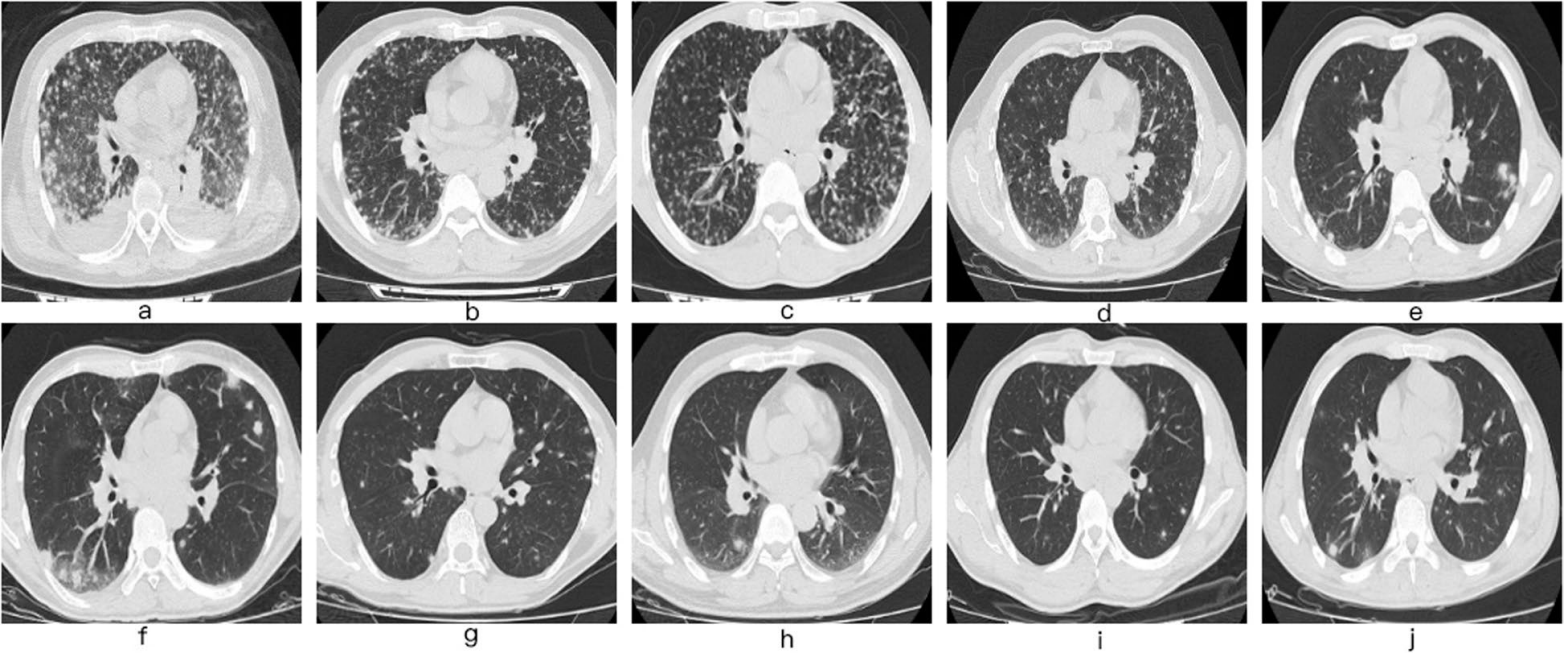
Manifestations of chest CT imaging of the 10 patients. The a-j refer to patients No.1-No.10 respectively
